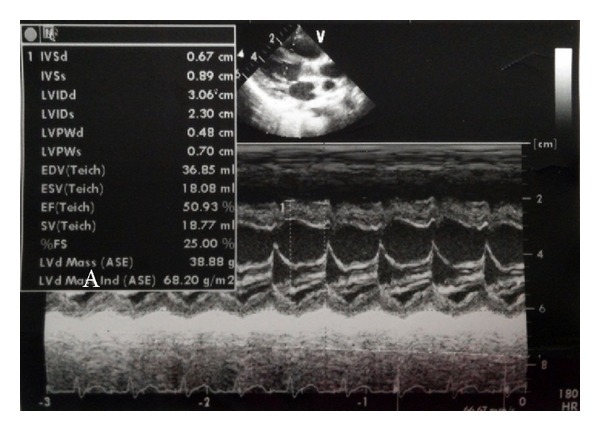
The echocardiographic findings on the second day of admission show decreased fractional shortening (25%) of left ventricle.
